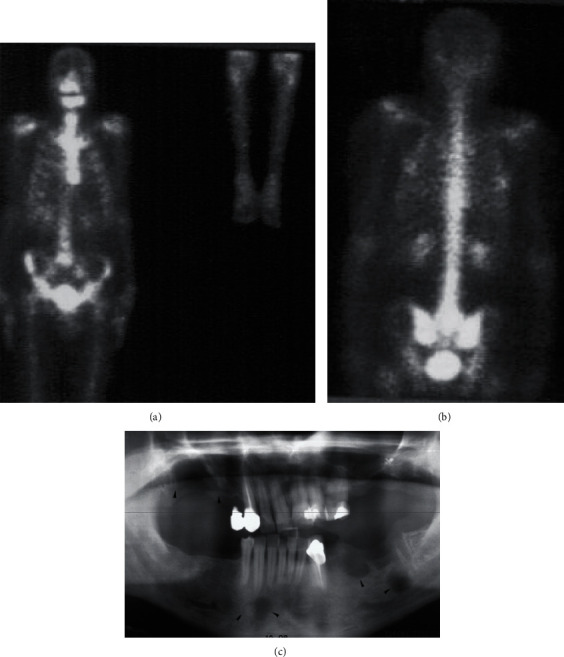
(a, b) Complete body scintigraphy with Te 99 revealing the only localization of LCH lesions in the jaws. (c) Panoramic radiograph after teeth extractions.
